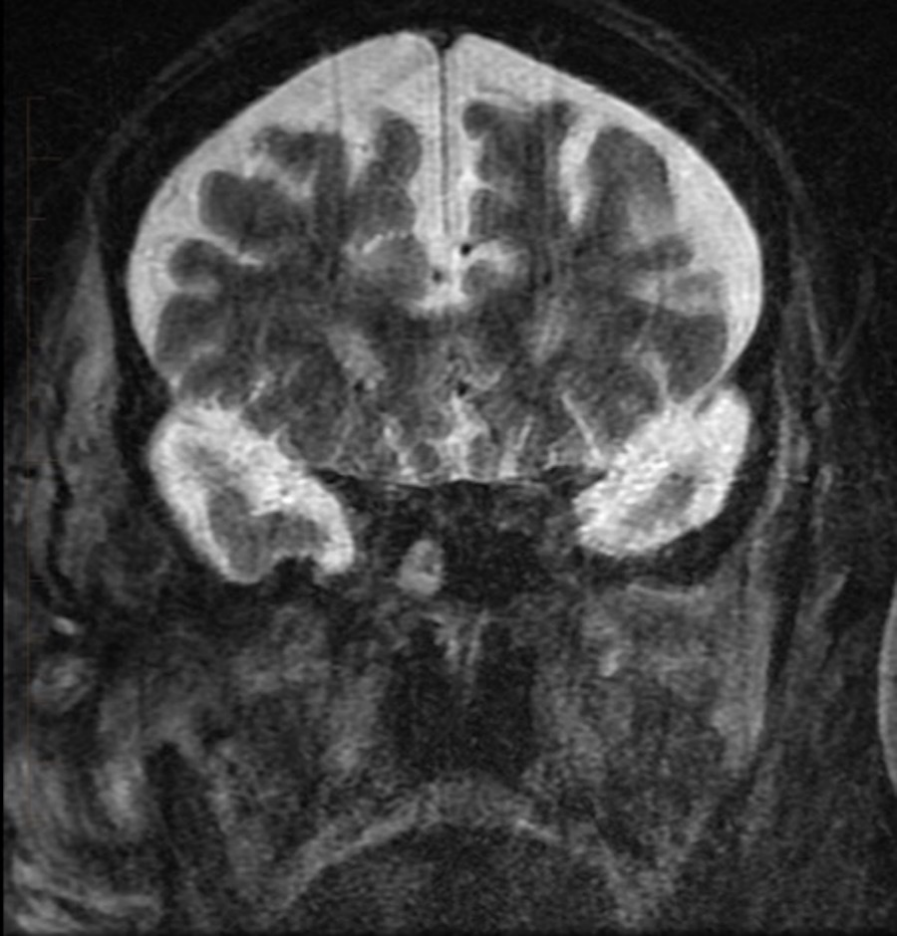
Follow-up coronal short tau inversion recovery magnetic resonance imaging five months after treatment demonstrating absence of the previously noted pseudoaneurysm and marked improvement of trans-spatial inflammatory changes.
